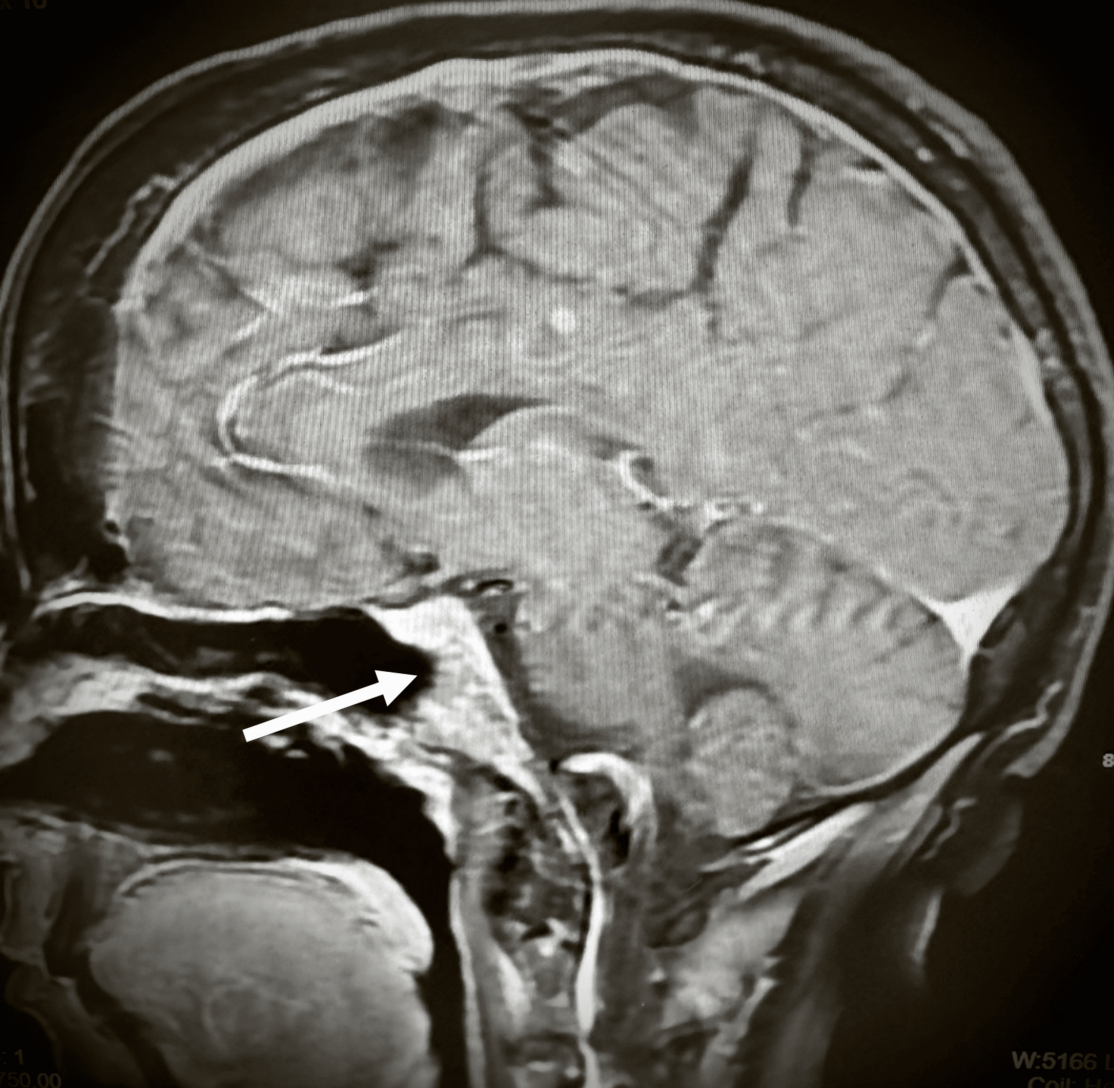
MRI of the head shows a 7 x 4 mm peripherally enhancing lesion within the clivus.
